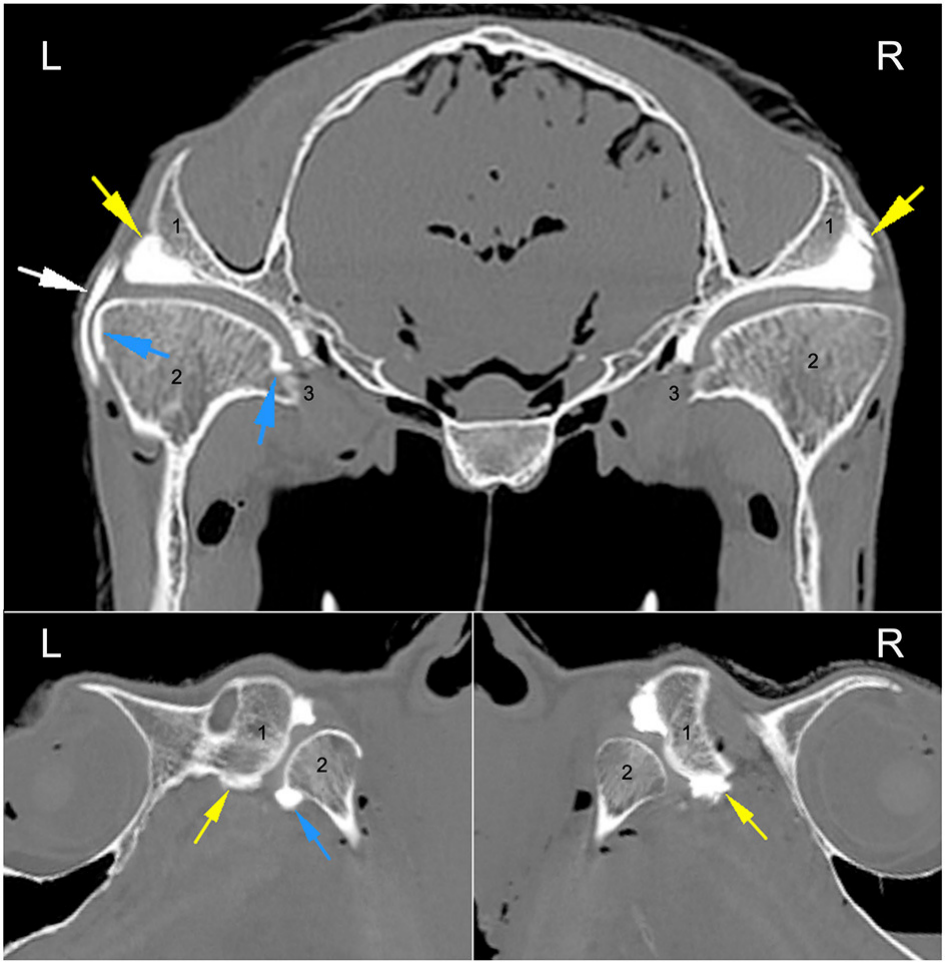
Axial and sagittal CT arthrographic images at the level of the TMJ of the 26-year-old Arabian gelding. Arrows indicate the presence of the radiopaque contrast material in the discotemporal joint (yellow), discomandibular joint (blue), and varying amounts in the subcutaneous tissues (white). L, left; R, right. 1, zygomatic process of the temporal bone; 2, mandibular condyle; 3, medial aspect of the mandibular condyle at the insertion of the pterygoid musculature bilaterally.
